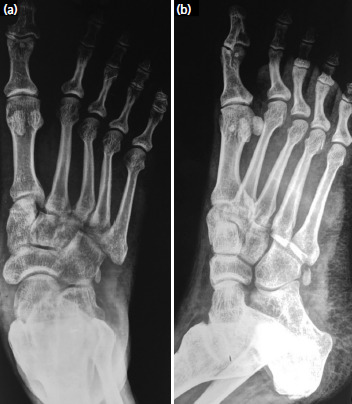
(a) Anteroposterior and (b) oblique radiographs of foot six weeks after closed reduction and immobilisation showing normally aligned 3rd MTP joint without subluxation/dislocation.
